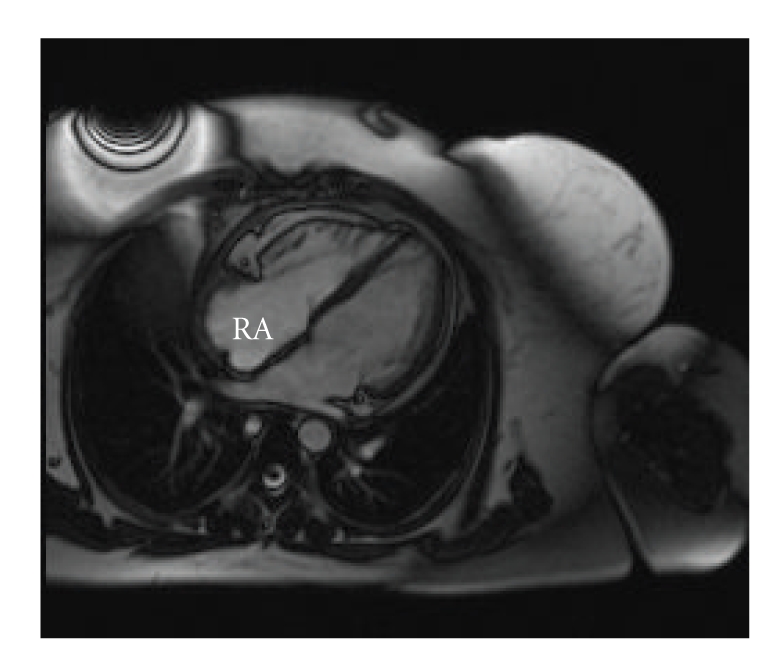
Cardiac MRI after chemotherapy of right atrial angiosarcoma showing significant resolution of the mass.
